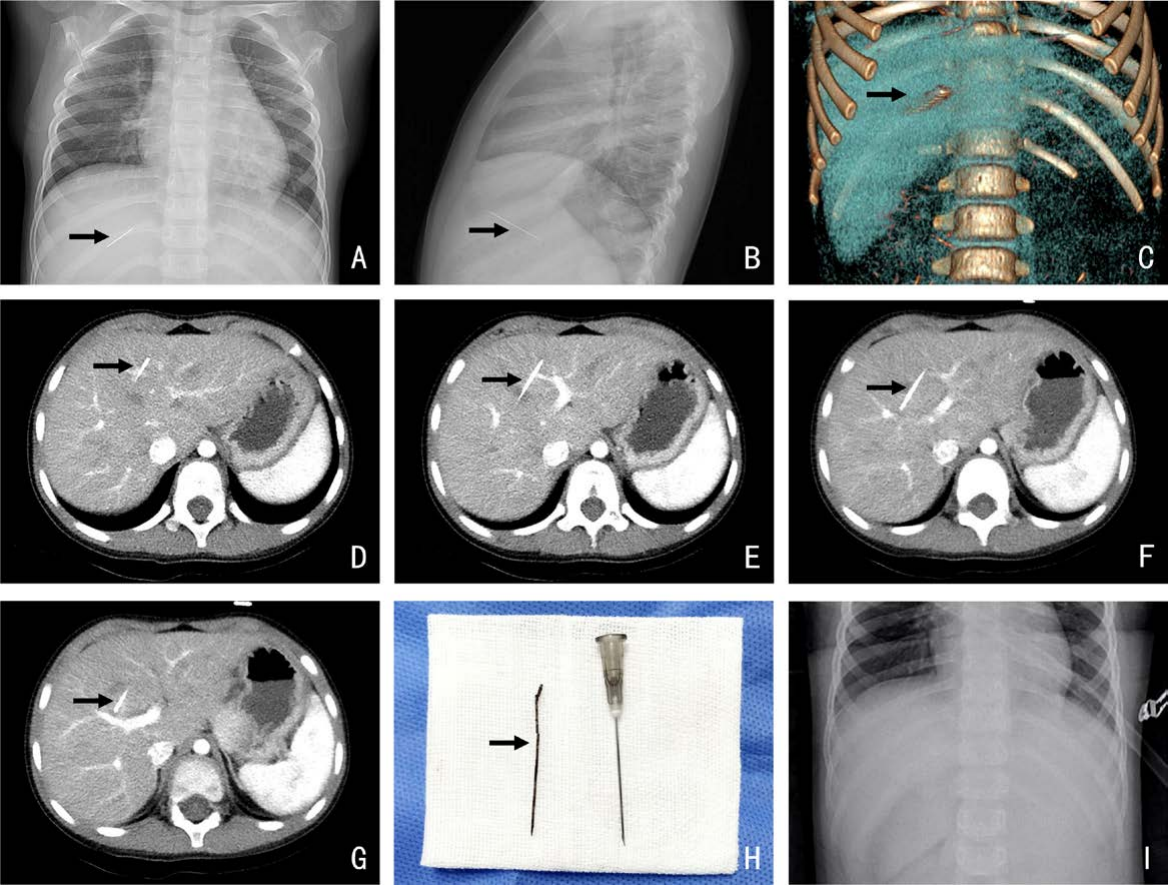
Preoperative and intraoperative images of case 1. Notes: The black arrow indicates FB. (A, B) Preoperative radiograph showing a high-density shadow in the hepatic region. (C) Preoperative CT reconstructed images showing a striated FB in the liver. (D, G) Contrast-enhanced CT showing the location of the striated FB in the liver before surgery. (H) The sewing needle is removed from the liver. (I) Intraoperative radiography showing that the FB had been removed.
